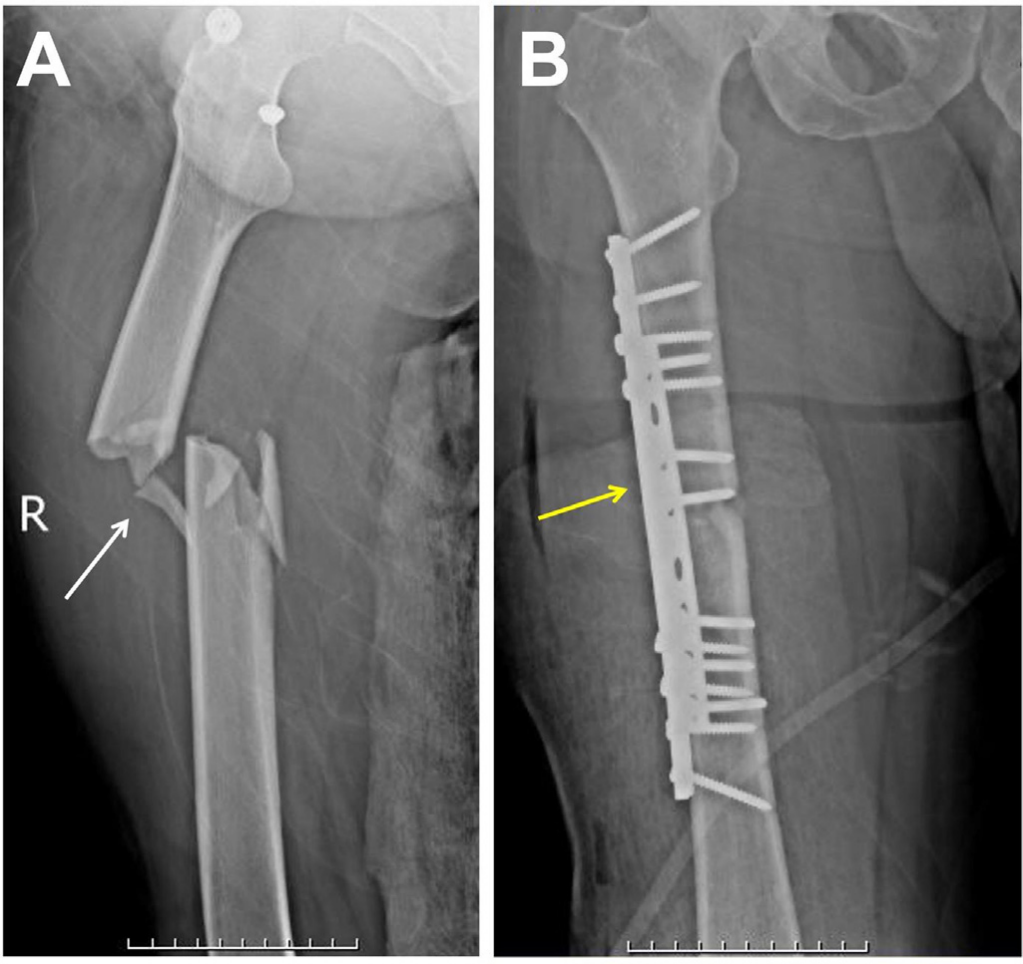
(A) Initial preoperative plain radiograph of the right femur (anteroposterior view) showing a femoral shaft fracture with medial displacement (white arrow). (B) Postoperative plain radiograph (anteroposterior view) showing open reduction and internal fixation of the right femur using 2 plates and screw fixation (yellow arrow).
